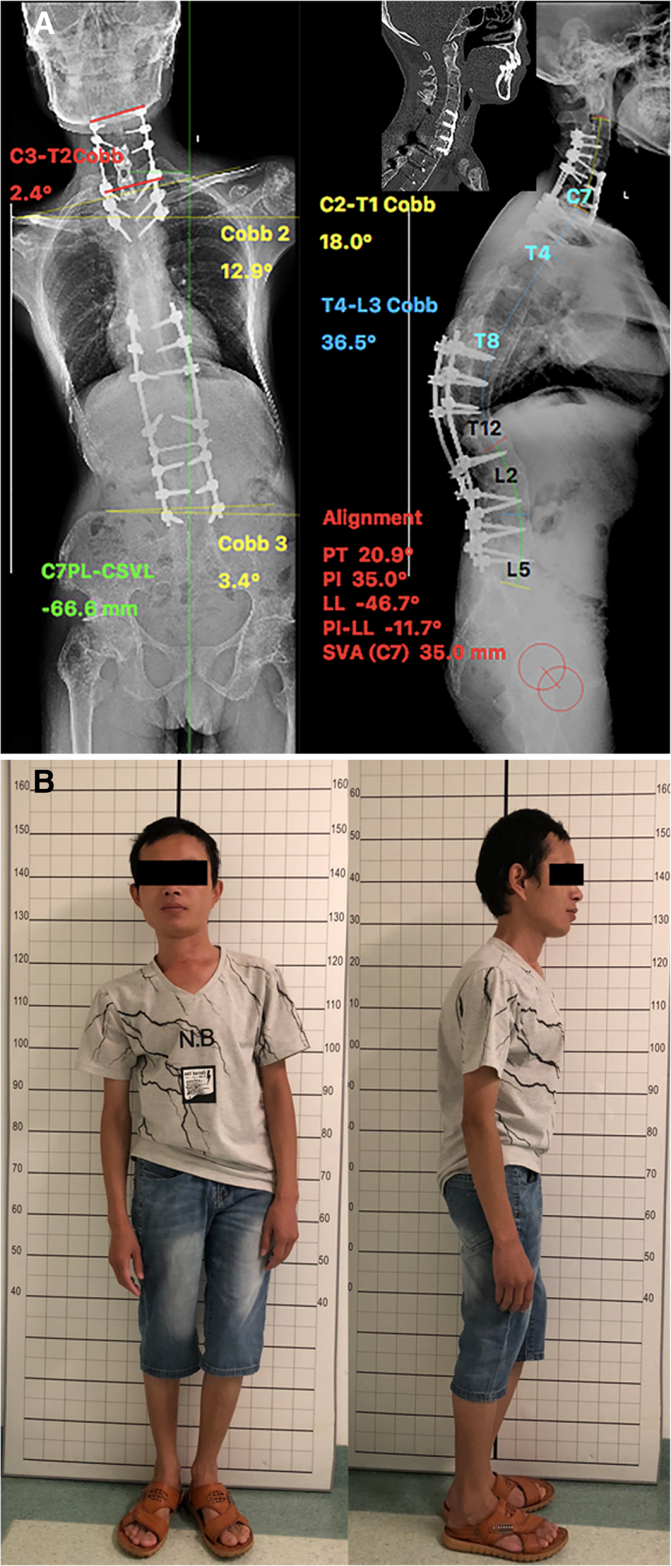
a Postoperative standing X-ray of the second-stage cervical osteotomy. The Cobb angle of C2–T1 in the sagittal plane was 18°. The Cobb angle of C3–T2 in the coronal plane was 2.4°. Three months after the second-stage cervical osteotomy, the CT scan of the spine showing bone healing of the osteotomy site. b Three months after the second-stage cervical osteotomy, the photographs show the patient with full correction of the spine. His CBVA was 3°
